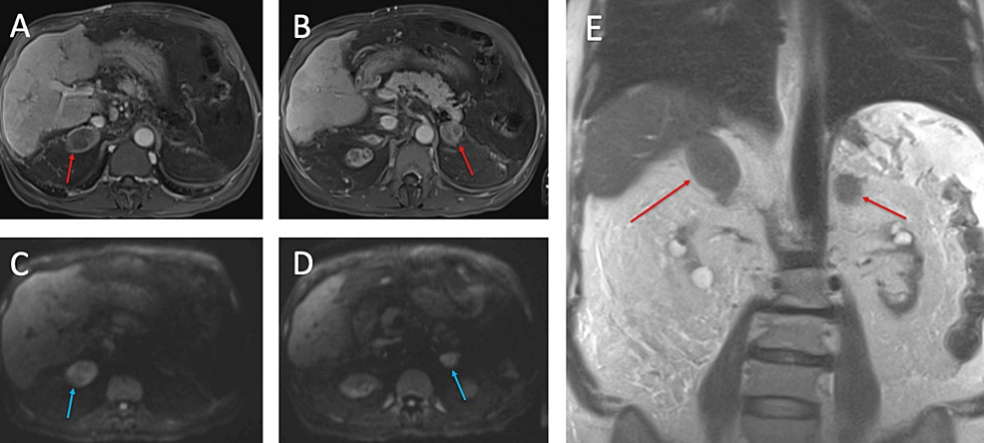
MRI of the abdomen with and without intravenous contrast showing bilateral necrotic adrenal glands Bilateral rim enhancing, centrally necrotic adrenal gland masses (red arrows), measuring (A) 4 cm on the right and (B) 2.9 cm on axial MRI with possible septations on the left. (C, D) The bilateral adrenal gland masses demonstrate diffusion restriction on axial MRI (blue arrows). (E) T2 haste sequence demonstrating T2 hypointense masses in bilateral adrenal glands on coronal MRI.
